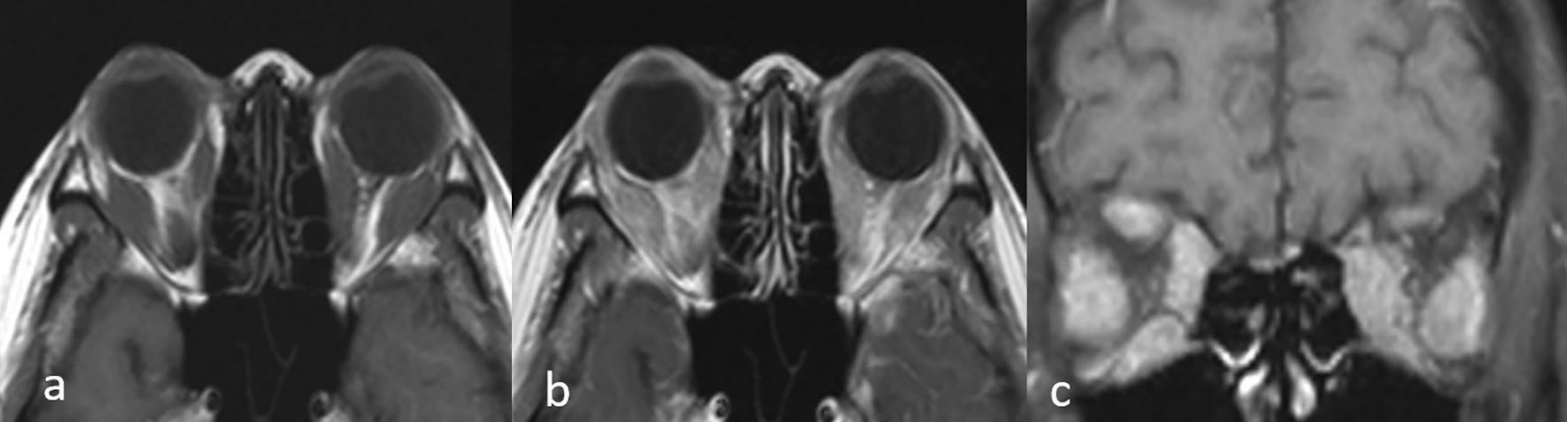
Multiple diffuse extraocular muscle metastases in a patient with solid tubular carcinoma of the breast. T1-weighted image a shows diffuse swelling of extraocular muscles. Contrast-enhanced T1-weighted image b and coronal contrast-enhanced T1-weighted image with fat suppression c show diffuse enhancement of the swollen extraocular muscles
